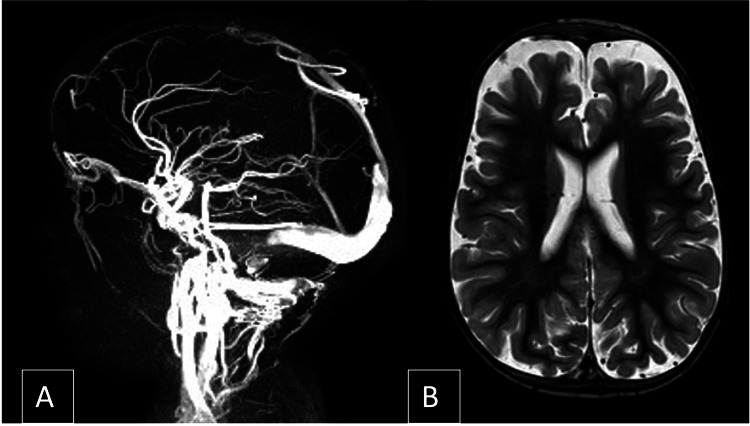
First brain MRI performed at 13 months old It was interpreted as being normal, apart from a dilated lateral sinus (A). The diagnosis of benign external hydrocephalus, a term used to define a rapid increase of head circumference in combination with increased subarachnoid space width (B), was made.
